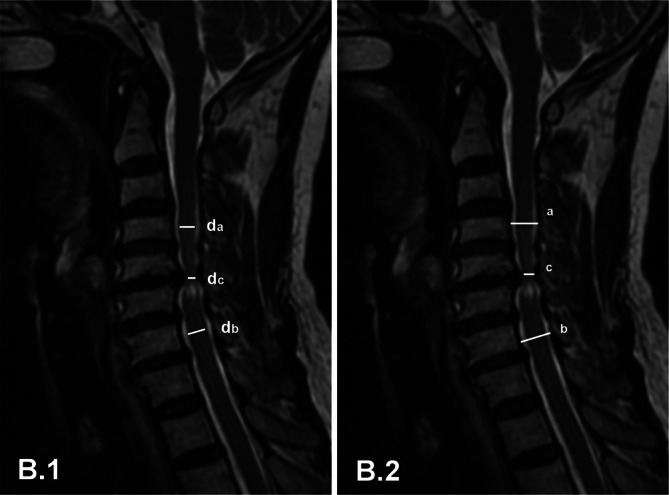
Compression ratio of the spinal cord and canal on sagittal MRI. (B.1) Measurement of the anteroposterior spinal cord diameter (dc) at the most severely compressed region. The diameters (da and db) are measured above and below the first normal vertebral segment, respectively. (B.2) Measurement of the anteroposterior spinal canal diameter (Dc) at the site of most severe stenosis on sagittal MRI. The diameters (Da and Db) are measured above and below the first normal vertebral segment, respectively.
